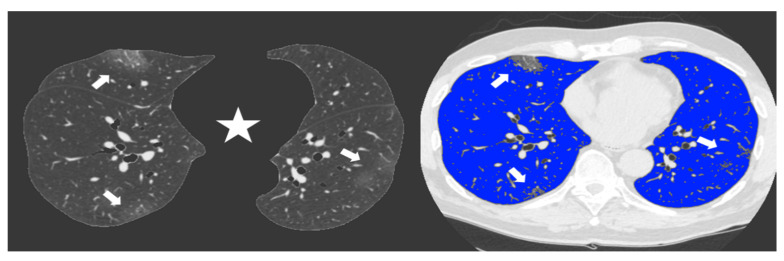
Non-enhanced axial Chest CT software reconstructions showing peripheral sub-pleural isolated GGOs (arrows). On the left the axial image automatically segmented by the software excluding all “non-lung” structures (mediastinum, upper airways and spine) (star) for visual quantification of infected parenchyma. On the right the same image highlighting in blue the well aerated lung and the COVID19 infection signs in grey. The whole infected volume assessed by software was 7% of the entire lung volume. The patient had good clinical course without need for invasive ventilation.
